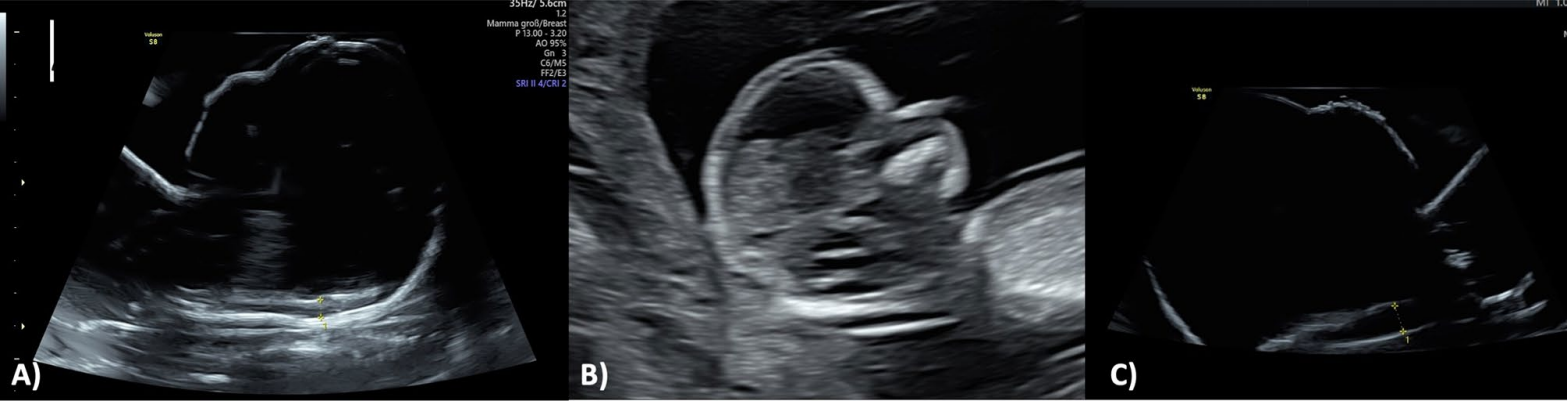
Comparative visualization of the physiological nuchal translucency (NT) in the fetal 3D model (A) and in the sonogram of the healthy fetus (13 + 0 weeks of gestation) (B) as well as the unphysiological fetal 3D model (C)
